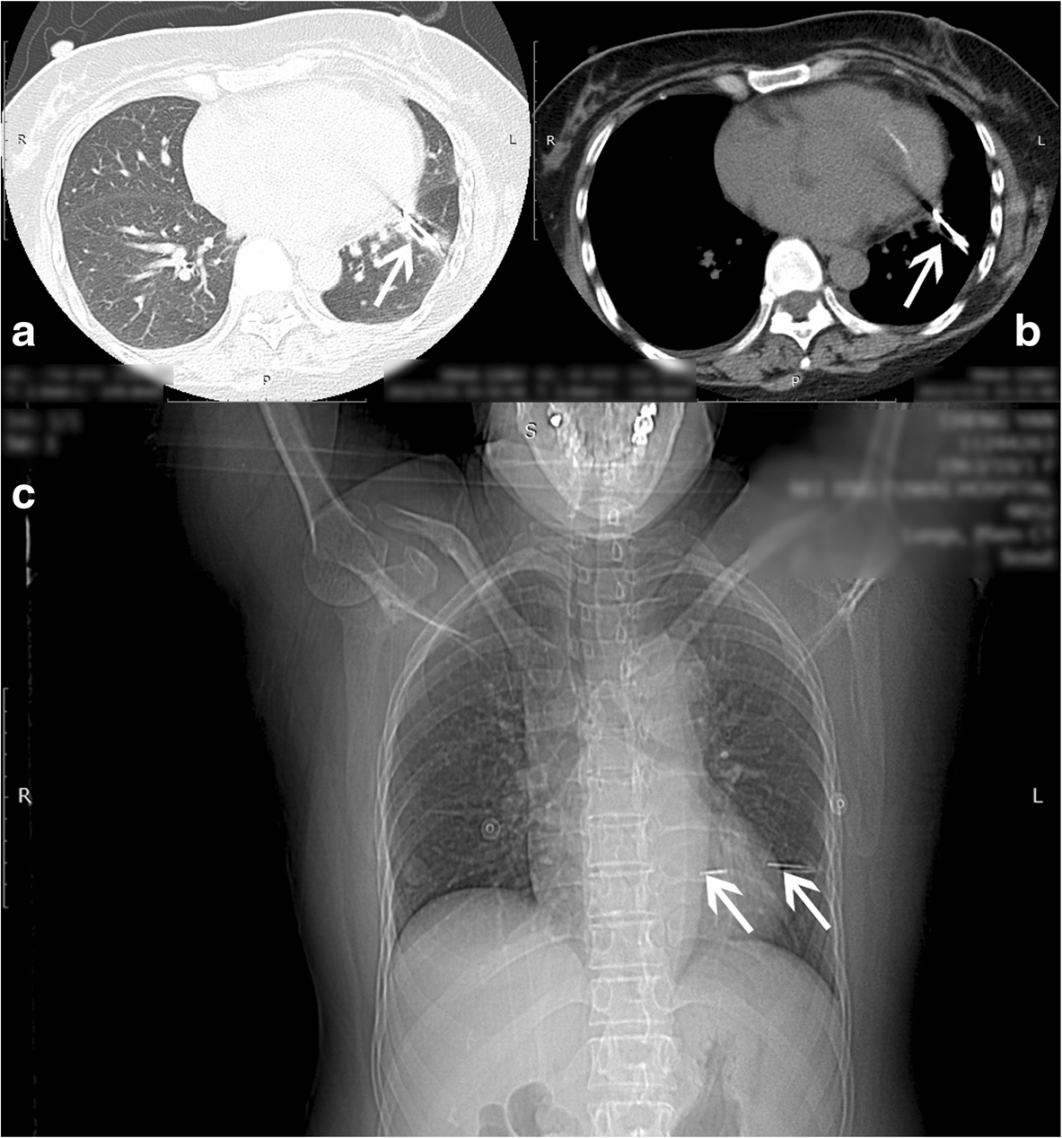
Chest computed tomographic images show the foreign body (*arrows*) inserted into the left lung and left ventricle. a The lung window. b The mediastinal window. c The whole chest
